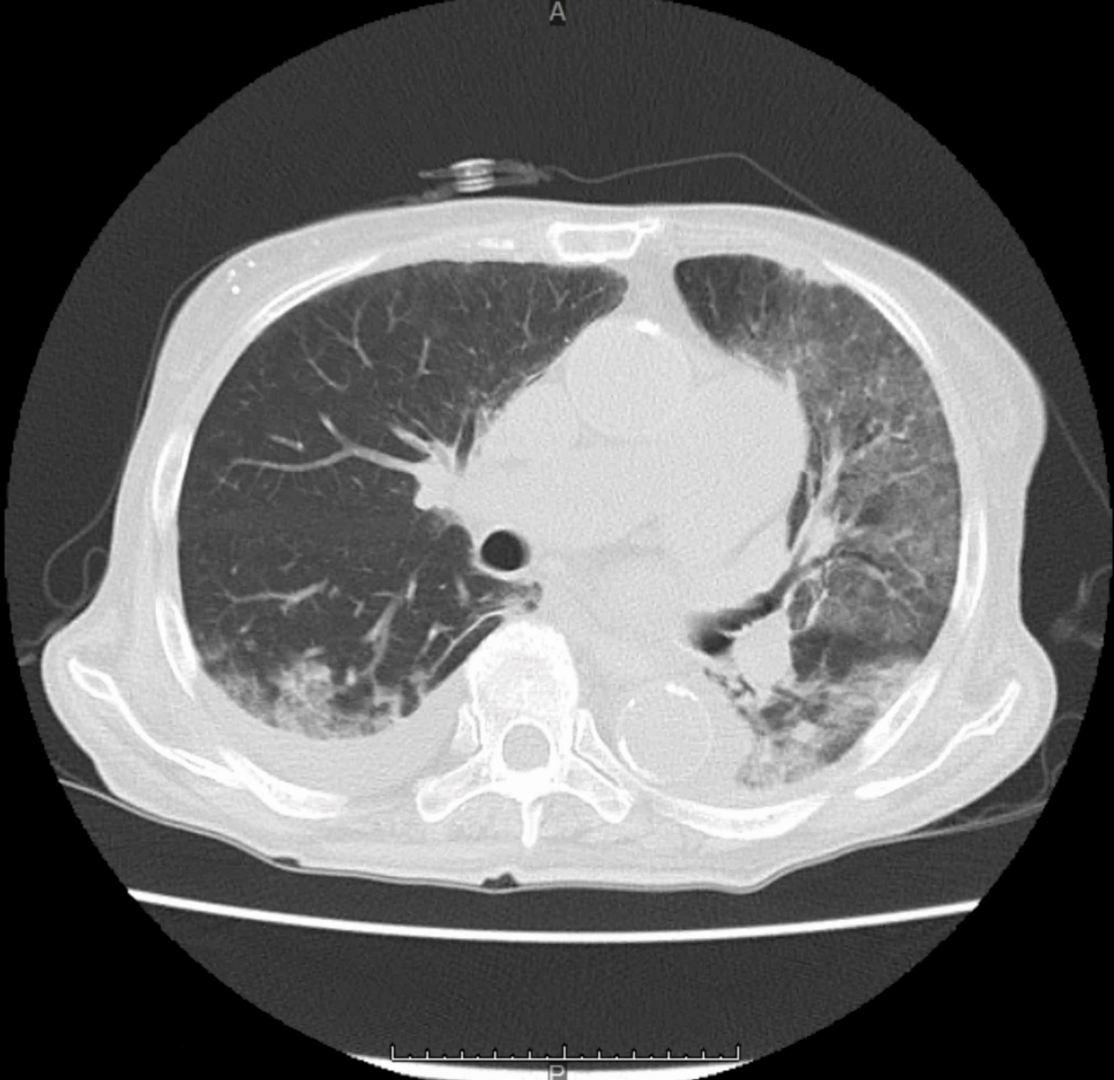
CT scan of the chest CT: computed tomography
